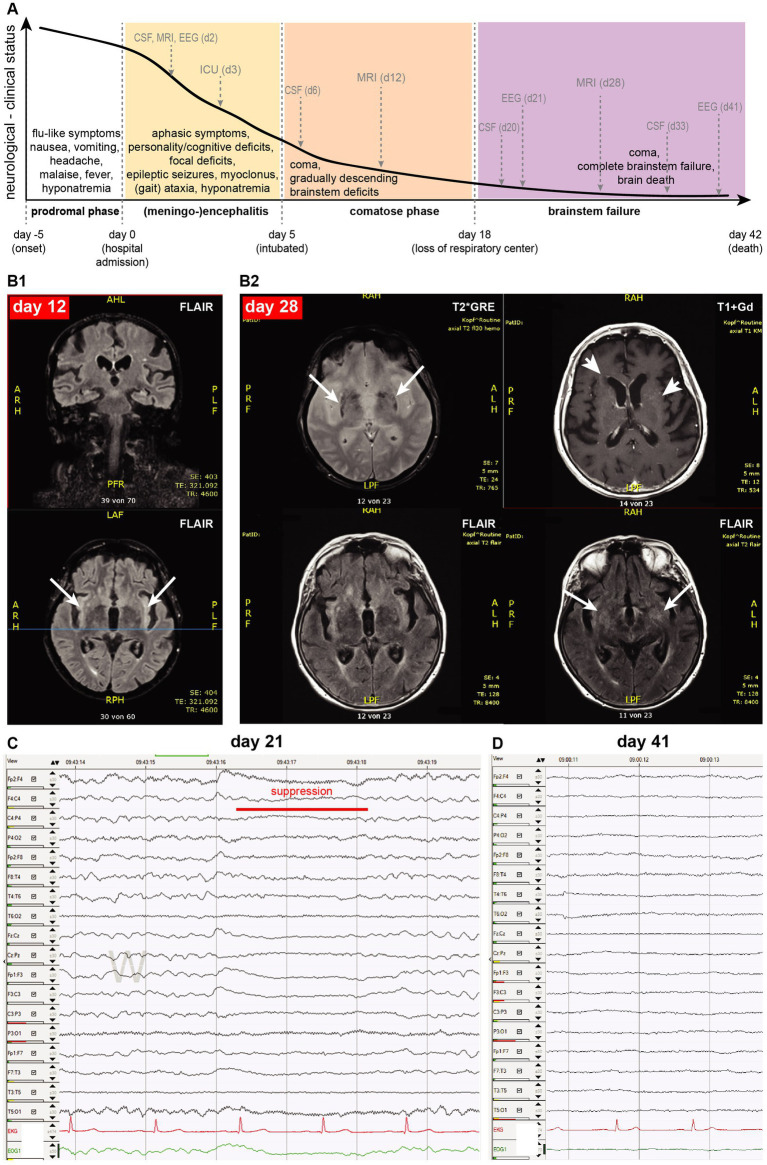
(A) Clinical course of the patient, shown as an arbitrary line of her neurological/clinical status decline (y-axis) against time (x-axis): grey arrows indicate running of main diagnostics as described in the text, dotted gray vertical lines indicate milestones of her disease course (onset, admission, intubation, and loss of respiratory center). (B1) The brain MRI on day 12 was mainly unremarkable, except for mild FLAIR signal increase in both insular cortexes (arrows). (B2) The MRI scan on day 28 showed age-related likely mineralization alterations in the basal ganglia (T2*GRE: arrows, present also in MRI of day 12, not shown here), diffuse and punctual gadolinium enhancement (T1 + Gd: arrowheads), and subtle expansion of increased FLAIR signal (FLAIR: arrows). (C) EEG on day 21 shows spontaneous burst suppression (red line) between theta-delta diffuse slowing, while the patient was clinically comatose. (D) On day 41, there is a complete absence of EEG-activity.
